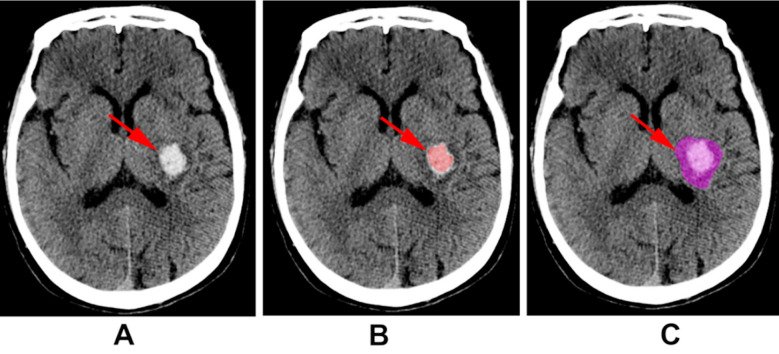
Images of head CT. A: primary image of cerebral hemorrhage. B: region of interest (ROI) of main hematoma and surrounding small hematoma and edema. C: ROI of main hematoma.
